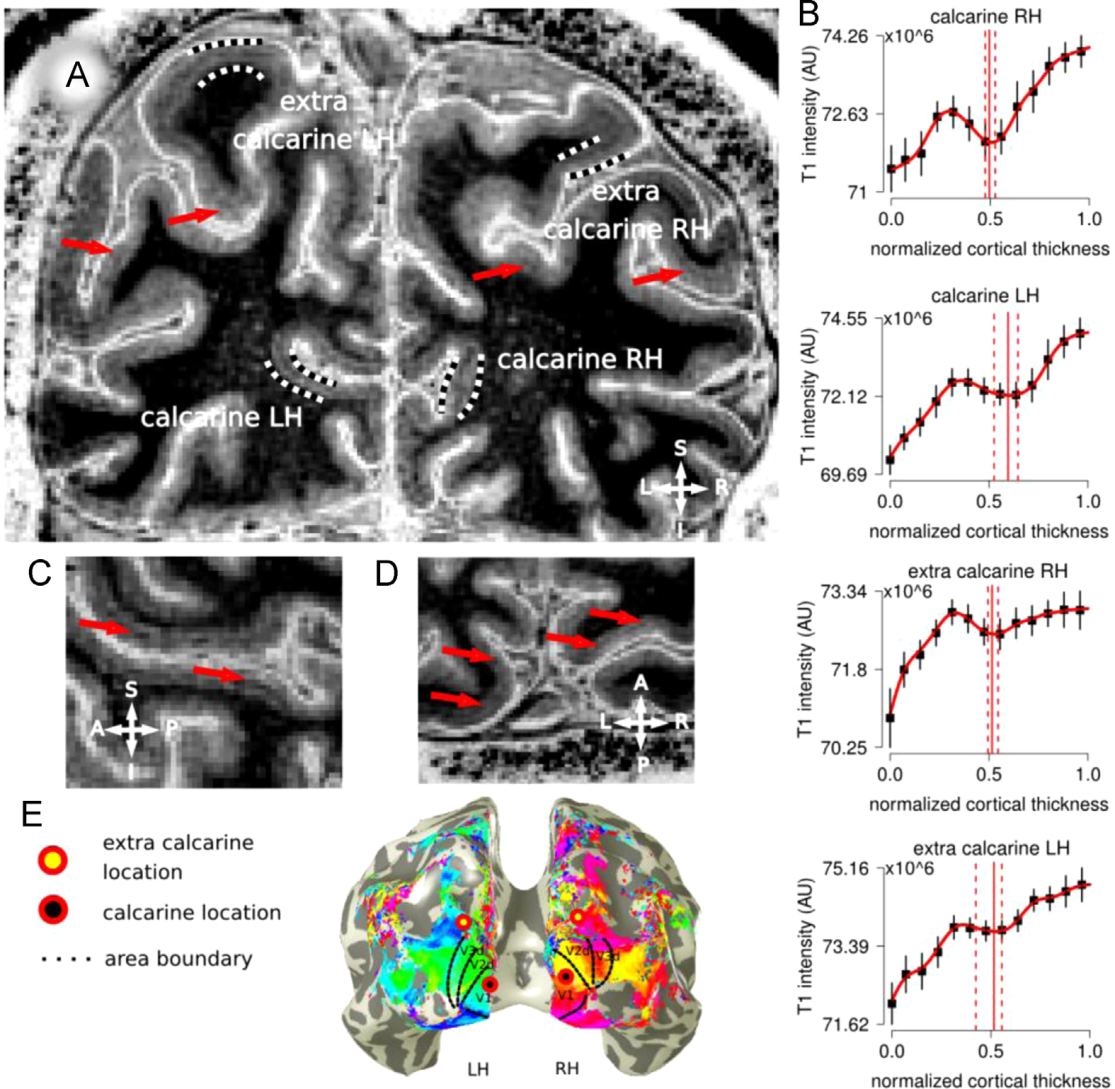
Panel A, in vivo T1-w image for one participant (P1). Locations in the calcarine cortex and extra-calcarine cortex are indicated in the image (black white dotted lines). Red arrows point to other locations where an hypo-intense band is visible around the middle of cortical thickness. Panel B, Laminar profiles are reported from the GM/WM border (0 on the *x*-axis) to the GM/CSF surface (1 on the *x*-axis) for each location. Error bars indicate the standard deviation over all profiles for each location, red lines in graphs represent median and bootstrapped 95% CI of the hypo-intense band location. A T1-w hypo-intense band was identified in more that 75% of the bootstrapped profiles (see ׳Laminar data analysis׳) in calcarine and extra-calcarine locations. Panel C, sagittal view; Panel D, axial view, centered on the calcarine; Panel E, early visual area retinotopic maps for participant 1, superimposed to the reconstructed whole brain 3D mesh from the conventional T1-w anatomical MRI. Areas V1 to V3 are reported. Black dots with a red surround represent calcarine locations projected to the mesh surface, yellow dots with a red surround represent extra-calcarine locations projected to the mesh surface. Extra-calcarine locations are well outside the functionally defined area V1. 3 The datasets for the other two participants follow (results from participant 1 are reported in [1]).
